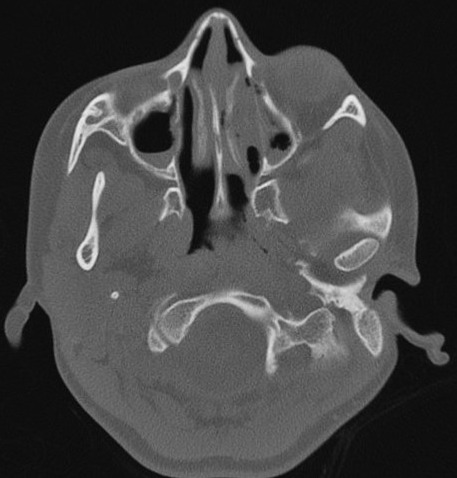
Sinus CT scan reveals maxillary sinusitis and filling of nasal pits mainly on the left side
